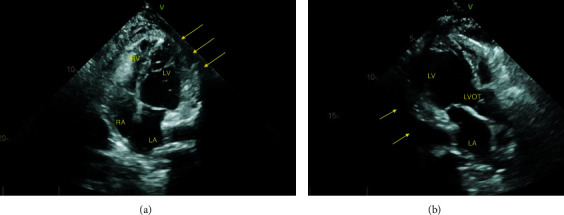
Transthoracic echocardiogram on day 1. The left ventricle has normal size. There is diffuse mild hypokinesis, LVEF is 45-50%. The right ventricular free wall appears thickened and echogenic. There is a small pericardial effusion. (a) Apical 4-chamber view and (b) parasternal long-axis view.
